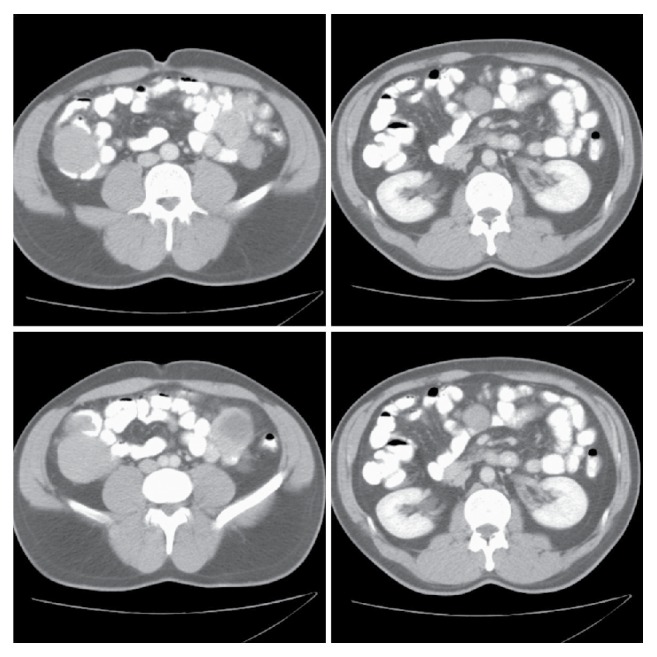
Computed tomography images of the lesions.
